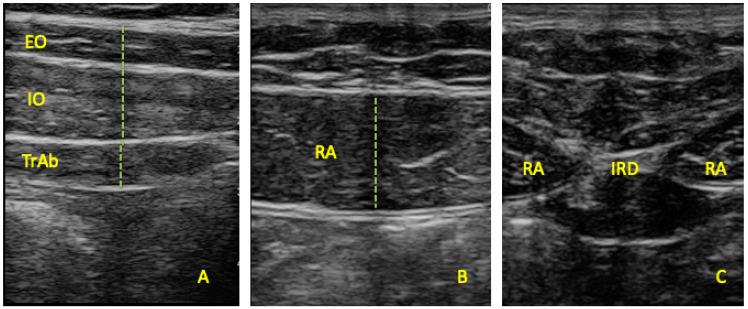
Ultrasound thickness assessments of the (A) EO, IO, TrAb, (B) RA, and (C) IRD.
